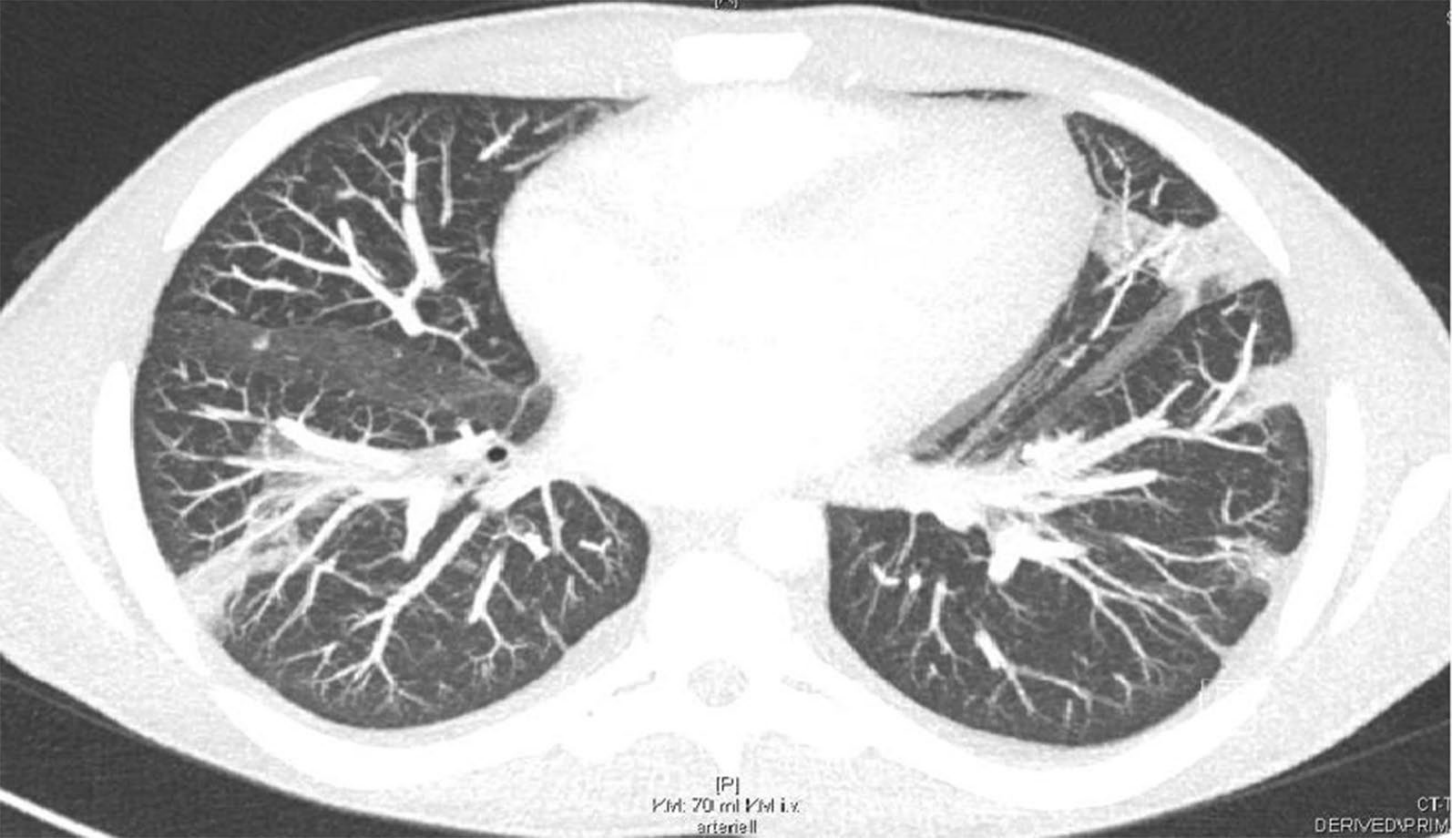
A follow-up thorax CT scan demonstrated fewer pneumonic infiltrates and septic pulmonary emboli
